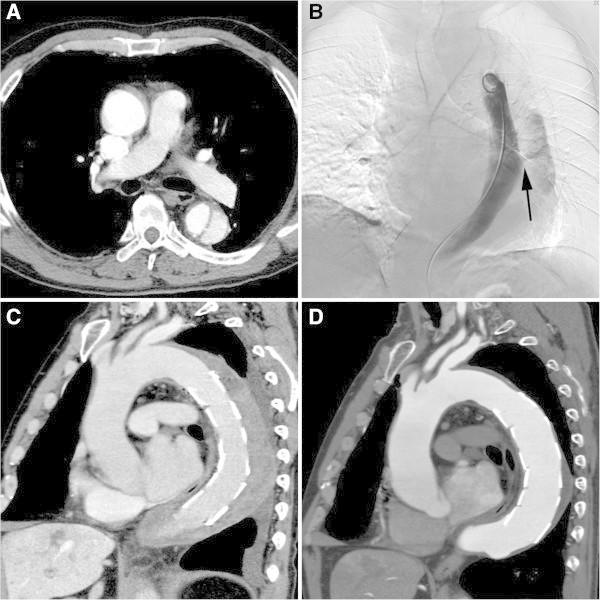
A 72-year-old male with type B acute AD. A. One month after onset, contrast-enhanced CT image shows progressive dilatation of the false lumen. B. Aortography shows the entry in the descending aorta (arrow). TEVAR (GORE TAG: 34-mm diameter & 20-cm length) was conducted to close the entry. C. One week after endovascular treatment, CT image shows thrombosed false lumen. D. Two months after endovascular treatment, contrast-enhanced CT image shows that the false lumen has markedly decreased.
